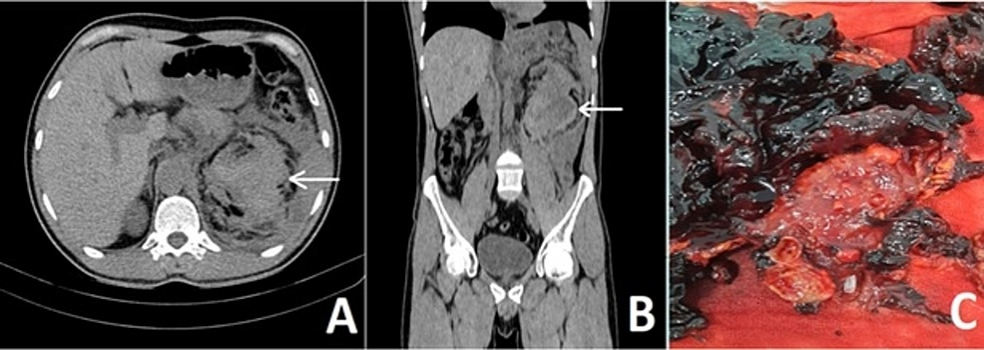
(A) Hematoma (arrow) can be seen at the posteroinferior of the left kidney in axial sections with a non-contrast abdominal CT. (B) Display of hematoma (arrow) in coronal sections. (C) Nephrectomy material. Multiple cortical cysts and solid nodular structures are seen on the kidney with the parenchymal disease.
